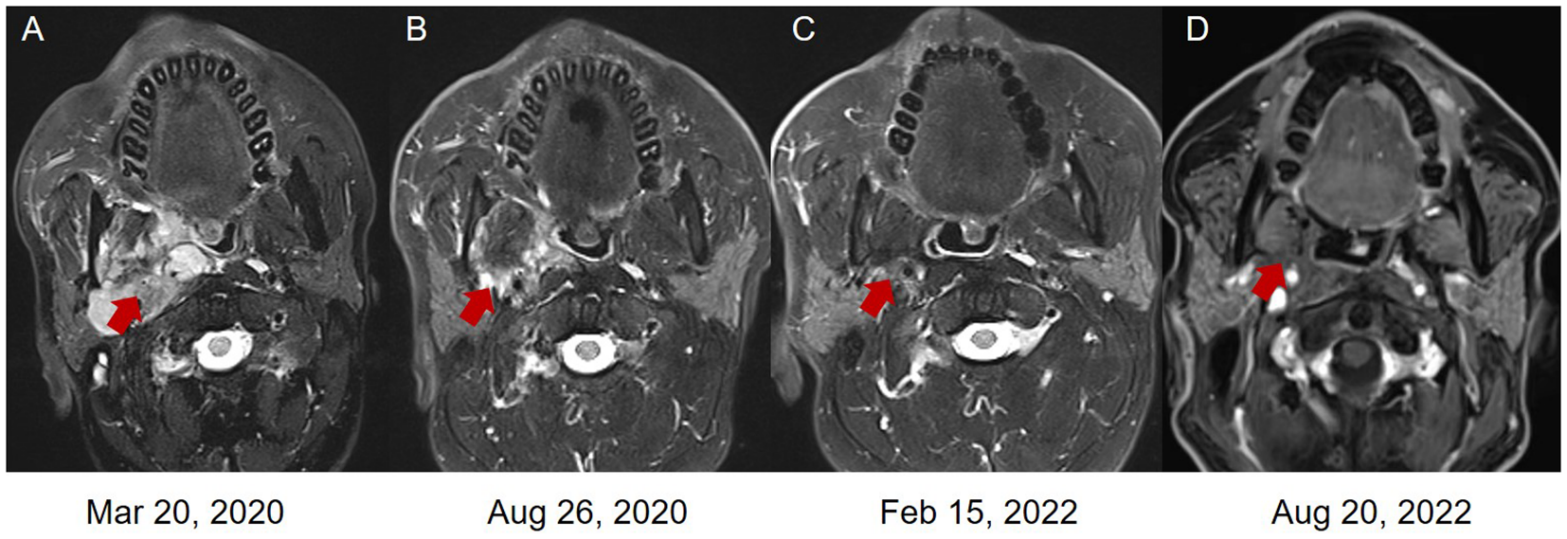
The magnetic resonance images (MRI) of the LGMS. (A) In March 2020, new multiple lymphatic metastases were observed. (B) In August 2020, the patient obtained a PR after the combined treatment of pembrolizumab and anlotinib. The patient obtained a PR (C) and CR (D) after the monotherapy of pembrolizumab.
